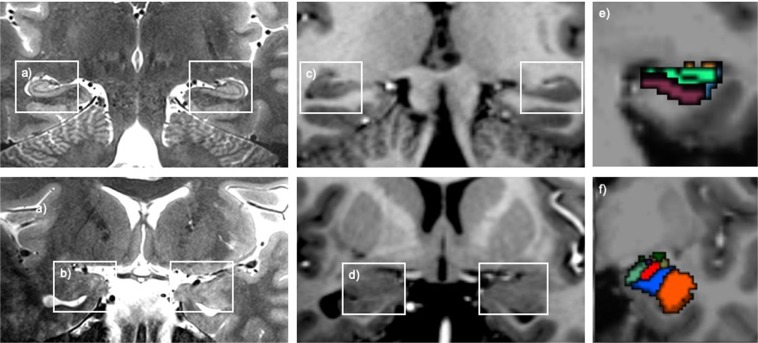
(a) T2-weighted images in the coronal orientation showing visibility of the hippocampus and (b) the amygdala at a resolution of 0.21 × 0.21 mm. (c) T1-weighted images in the coronal orientation showing visibility of the hippocampus and (d) the amygdala at an isotropic resolution of 0.7 mm. (e) Example coronal slice of the hippocampal subfield segmentation and (f) the amygdala nuclei segmentation.
